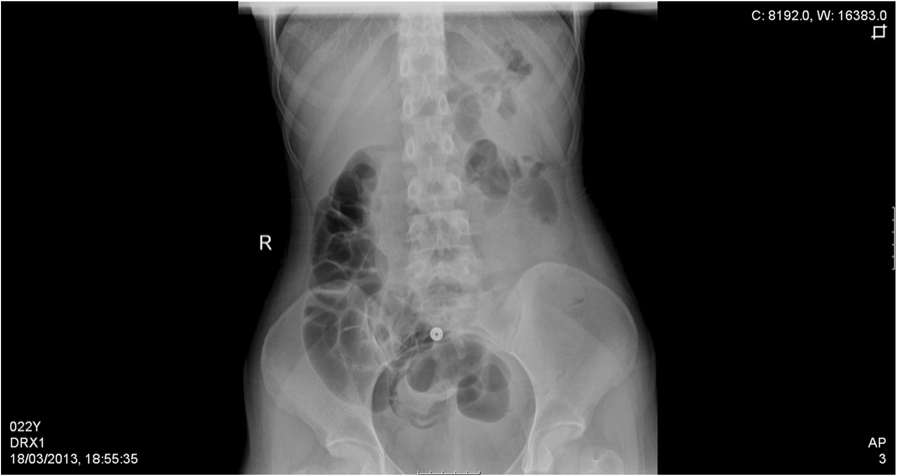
Plain abdominal supine radiograph revealing small bowel obstructive pattern with paucity of gas in colon.
